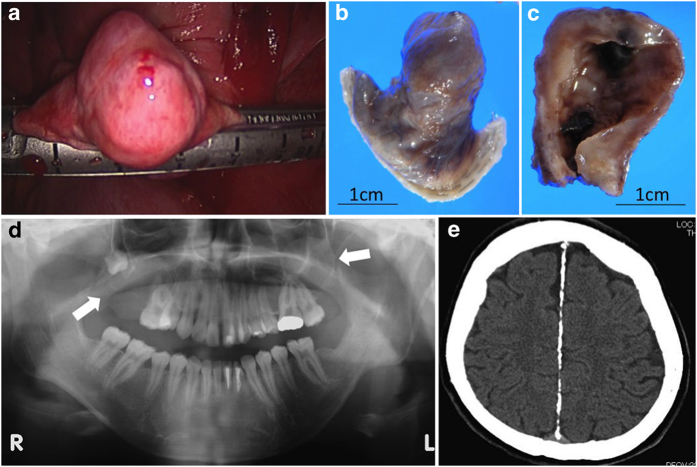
Intraoperative finding of the pleura and cystic lesion (a). Gross appearance of the resected cystic lesion with smooth surface (b) and the cut section with unevenly thickened cyst wall (c). An orthopantomogram showing cystic lesions in the mandibular region (white arrows) (d). Brain-computed tomography showing intracranial ectopic calcifications of the falx cerebri (e). L, left; R, right.
